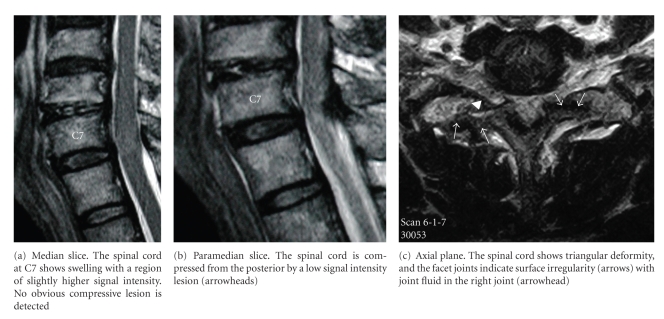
MRI on T2-weighted image.
